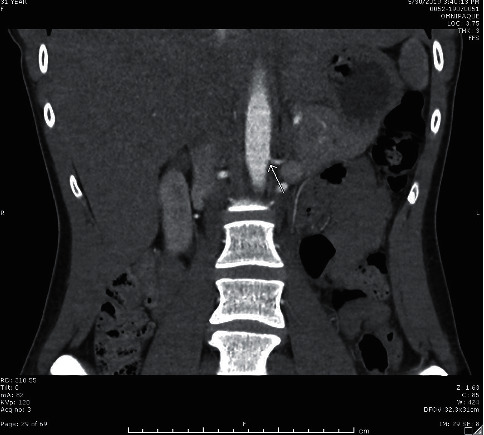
Coronal image from renal CT with contrast showing the area of ostial stenosis of left accessory renal artery.
